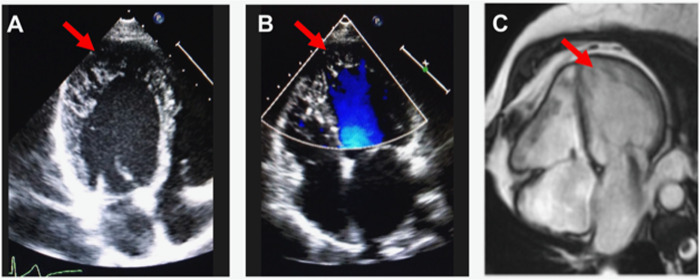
Echocardiogram and cardiac magnetic resonance imaging in LVNC. Left ventricular non-compaction from a representative subject from the study, on a, 2D-transthoracic echocardiogram, b, 2D-transthoracic echocardiogram with colour Doppler flow, and c, cardiac magnetic resonance imaging. Red arrows indicate regions of non-compaction.
